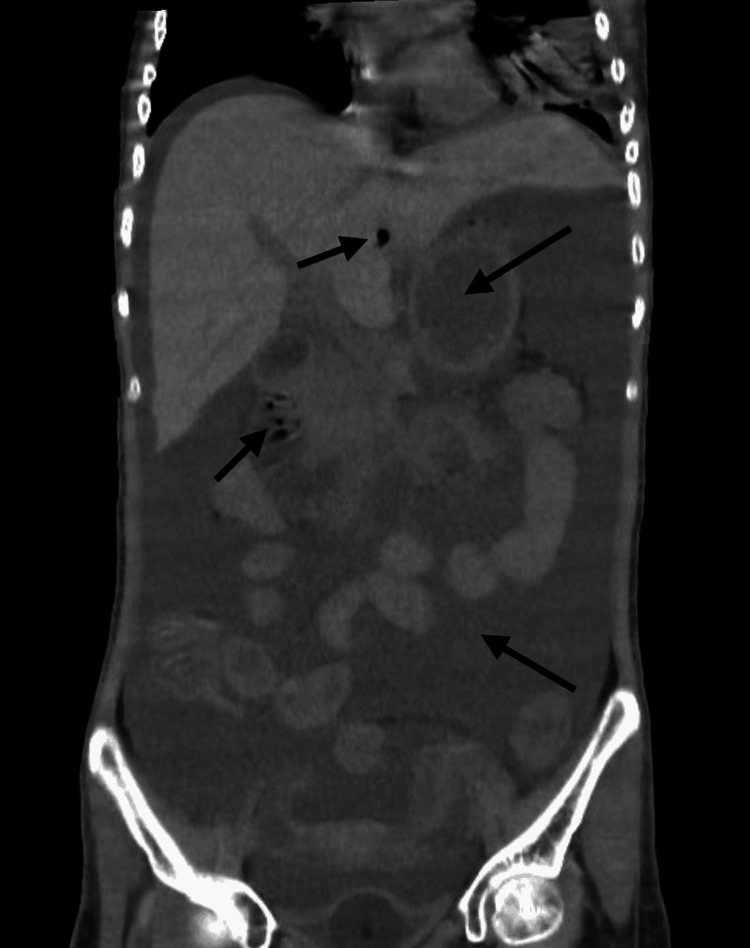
Preoperative abdominal CT on the day of perforation, approximately 16 weeks after the initial presentation, showing pneumoperitoneum and free fluid The arrows indicate foci of free intraperitoneal air (pneumoperitoneum), a discrete sub-diaphragmatic gas bubble and adjacent mottled extraluminal gas locules, the distended gastric chamber, and free intraperitoneal fluid (representative of the large-volume free fluid present). Bilateral pleural effusions (left greater than right) with left basal consolidation were also noted. CT, computed tomography.
